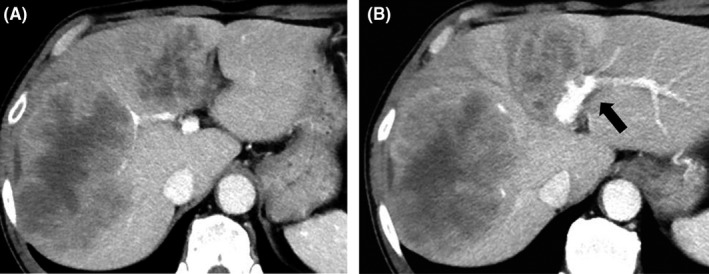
Initial enhanced computed tomography images. A, Multiple low‐density tumors with enhanced margins in the liver. B, The tumors invading the umbilical portion (arrow)
